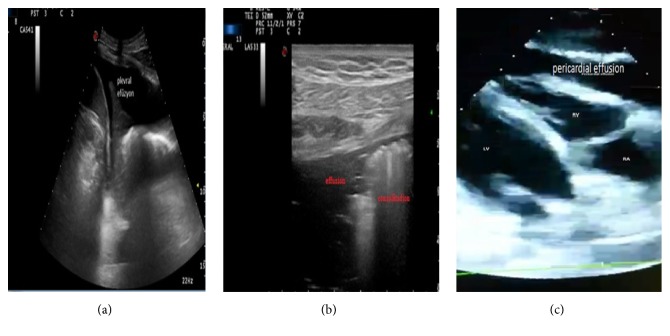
Some of the ultrasonographic pathologic views obtained during the study. (a) Pleural effusion view in the left pleural area in the left upper quadrant ultrasonography. (b) Pneumonic consolidation and parapneumonic effusion view in thoracic ultrasonography. (c) View of pericardial effusion compressing the left ventricle on the parasternal scan in the cardiac ultrasound.
